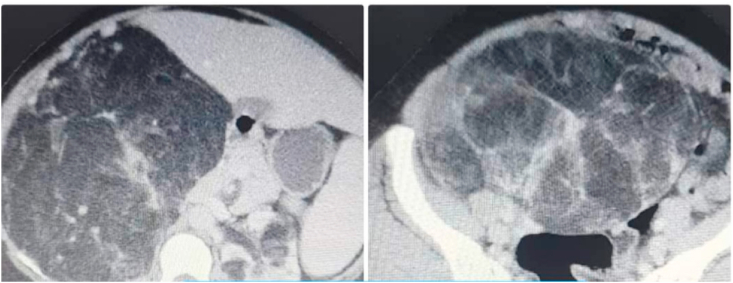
Computed tomography shows right renal giant mass.
